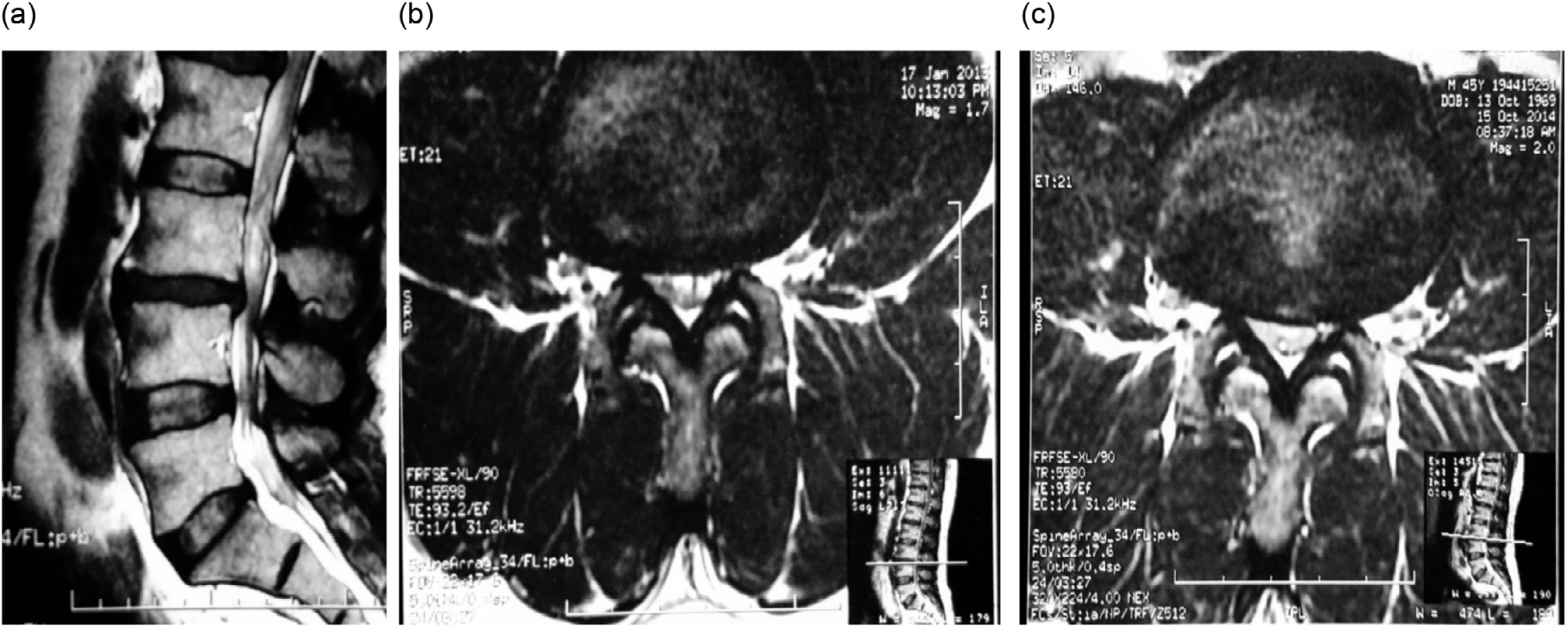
MRI lumbar spine; (a) sagittal view shows L3-4 disc degeneration, (b) axial view of the same patient shows atrophied multifidus on the same level of L3-4 disc degeneration, (c) increased cross-sectional area of LMF after 12 months of local intramuscular PLRP injection.LMF muscle atrophy is identified by a decrease in the muscle size and deposition of fat and connective tissue, which shows a high signal intensity on the fast spin echo T2-weighted images used.
